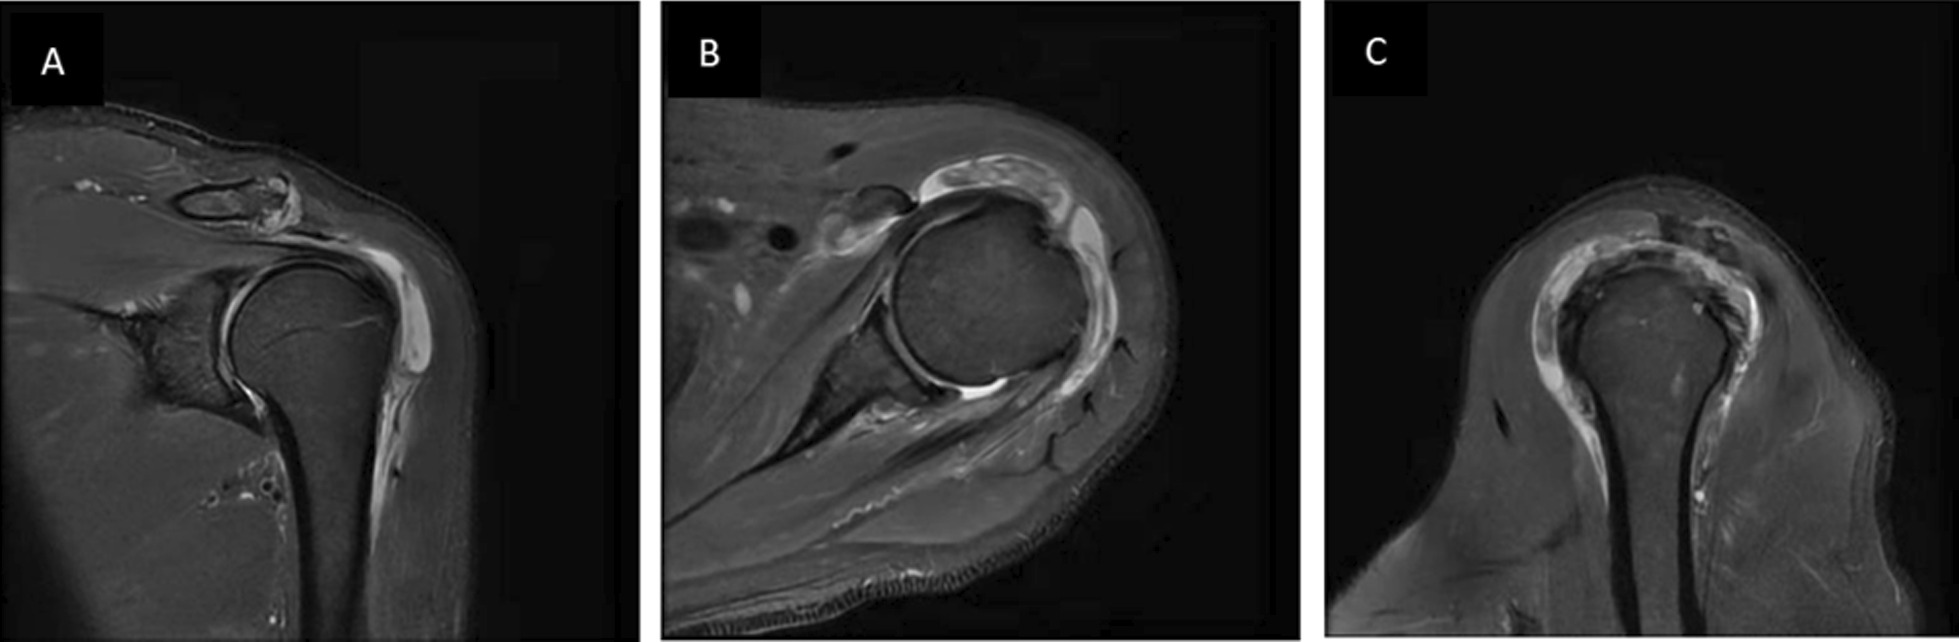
A 63-year-old male patient who underwent shoulder joint MRI and showed no obvious signs of soft tissue invasion around tophi. A T2-FS coronal, B T2-FS transverse, C T2-FS sagittal
